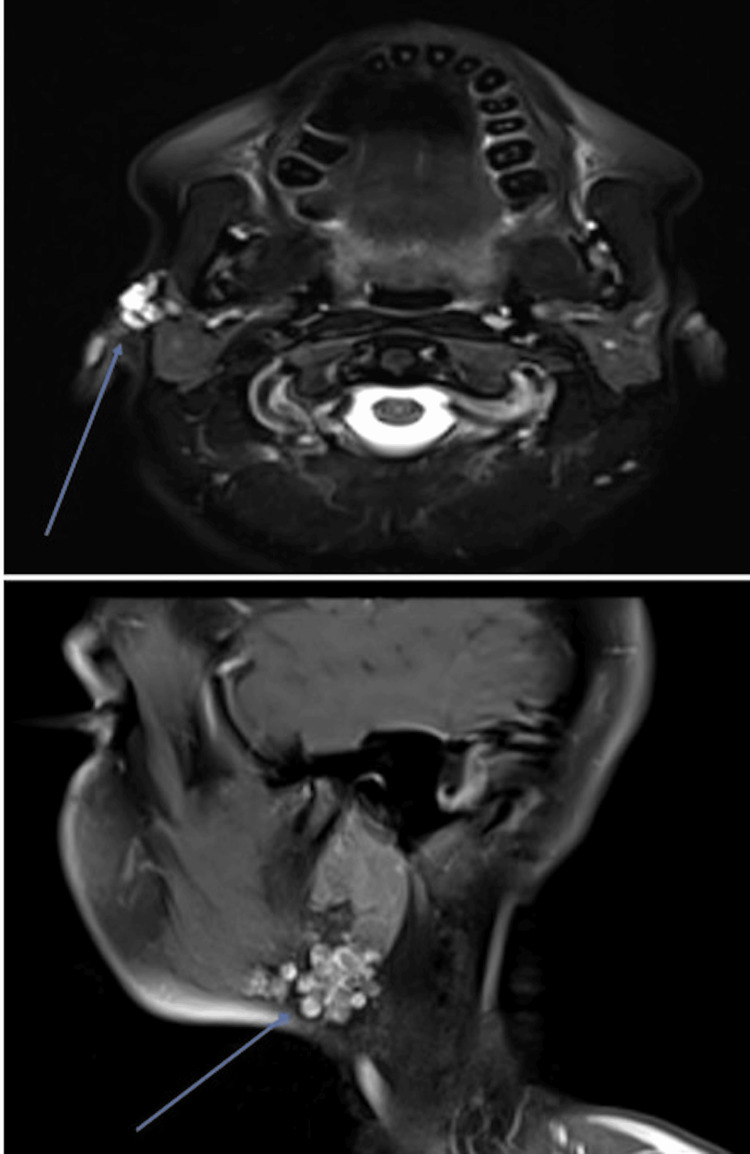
Axial and sagittal MRI images demonstrating the multifocal subcutaneous lesions
